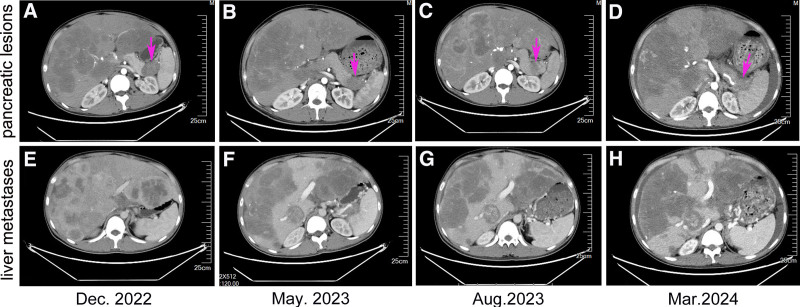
Enhanced CT imaging changes of pancreatic and liver during drug therapy. (A and E) Pancreatic and liver images evaluation before treatment: progressive disease (pink arrows: 4.1 cm in maximum diameter). (B and F) Pancreatic and liver images evaluation after treatment with penpulimab and anlotinib for 4 cycles: stable disease (pink arrows: 3.4 cm in maximum diameter). (C and G) Pancreatic and liver images evaluation after treatment of penpulimab combined with anlotinib for 8 cycles: stable disease (pink arrows: 2.3 cm in maximum diameter). (D and H) Pancreatic and liver images evaluation in March 2024, the lesions were enlarged and increased: progressive disease (pink arrows: 2.9 cm in maximum diameter). CT = computed tomography.
